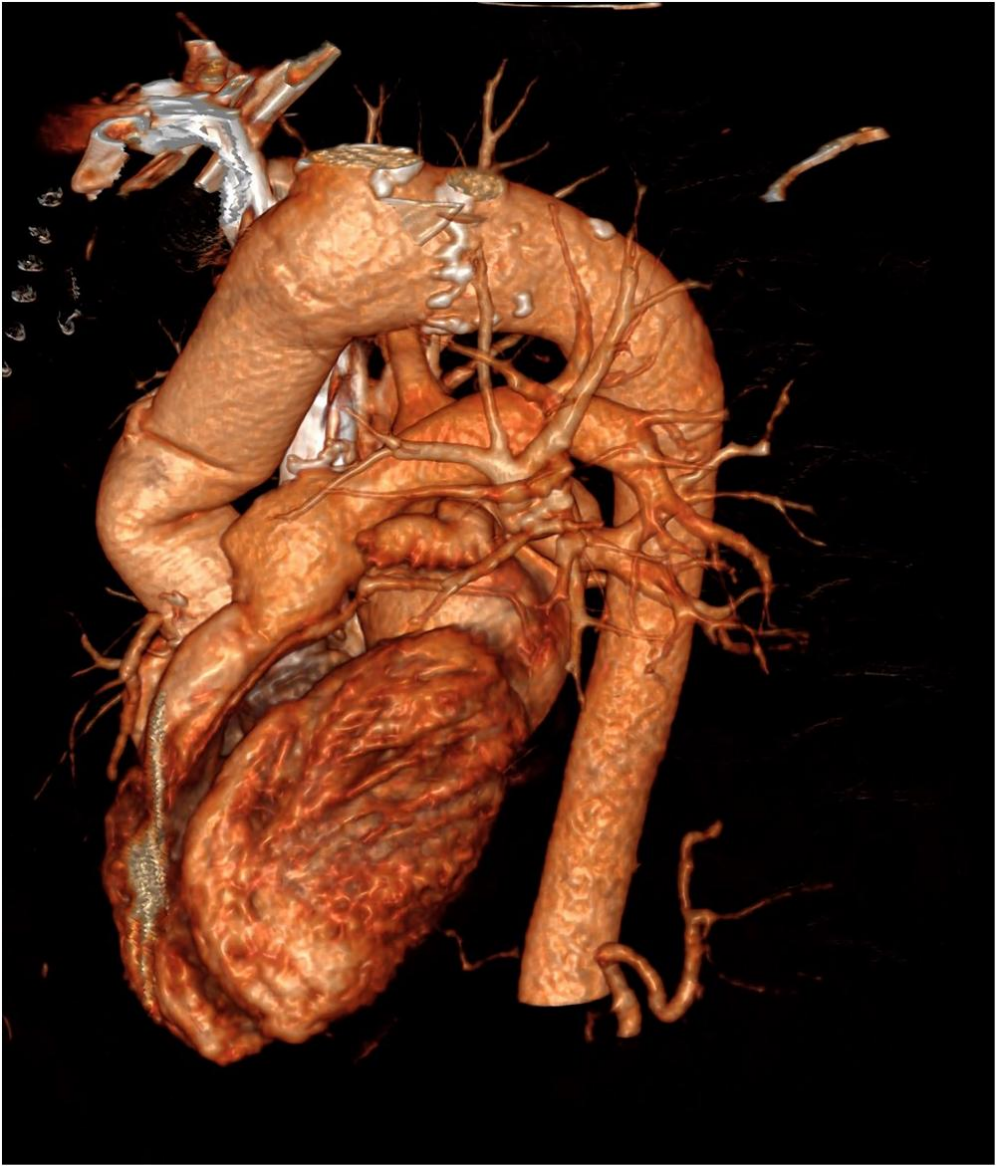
3D CT angiography reconstruction: post-surgical imaging.
